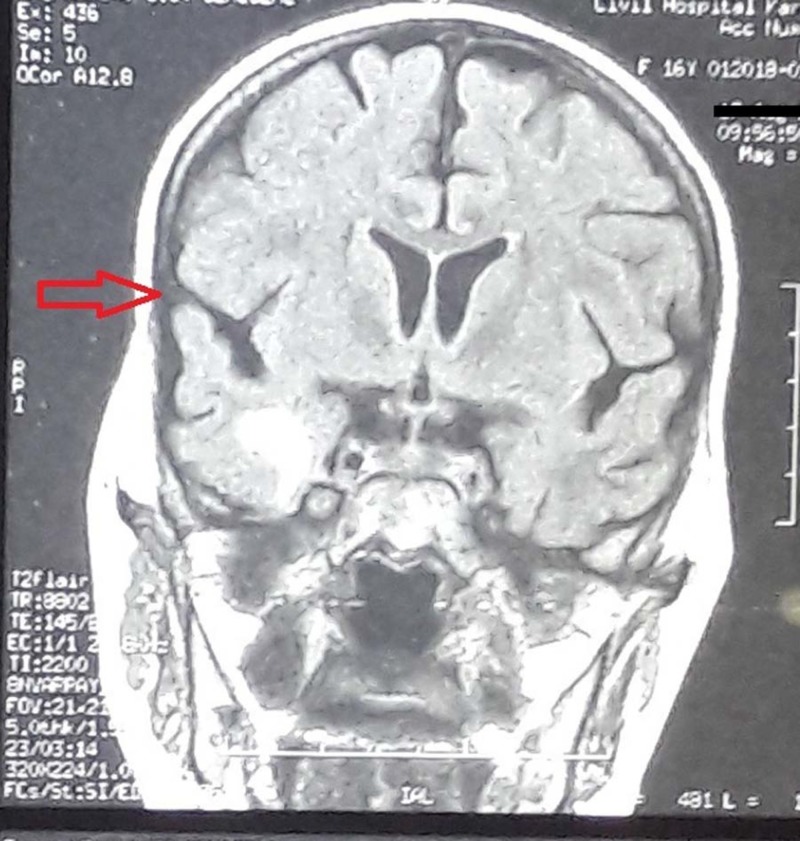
Brain MRI (coronal section) T1 weighted image showing a hypointense signal in the right temporal lobe consistent with intracranial extension of invasive fungal sinusitis.
